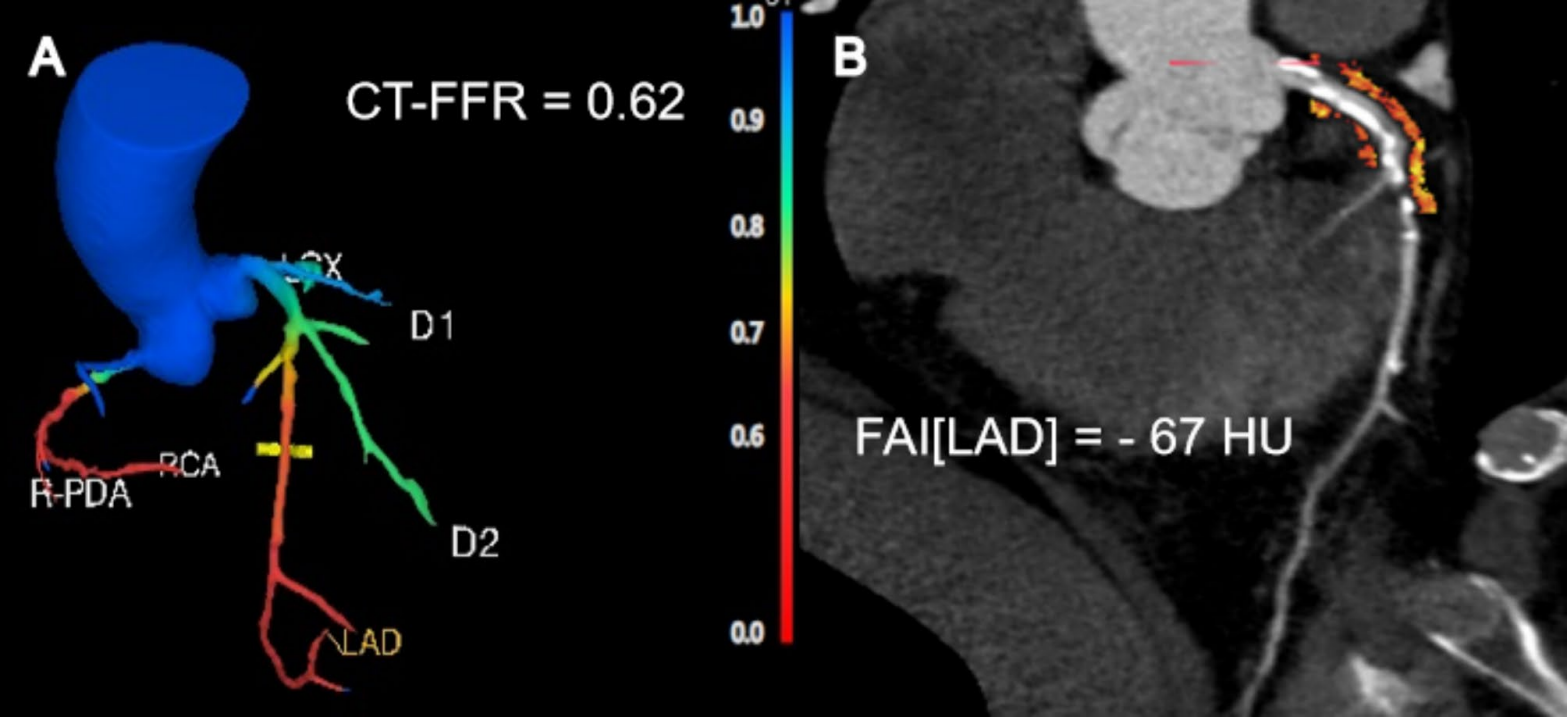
A representative case of CT-FFR and FAI assessment. (A) Three-dimensional color-coded mesh reveals hemodynamically significant lesions in the LAD, RCA, and PDA (CT-FFR = 0.62, CT-FFR ≤ 0.80 is considered positive). (B) Perivascular FAI phenotyping from CCTA in the proximal 0–40 mm of the LAD (FAI[LAD] = − 67 HU, FAI ≥ 70.1 is considered positive). CT-FFR, CT-derived fractional flow reserve; FAI, fat attenuation index; LAD, left anterior descending artery; RCA, right coronary artery; PDA, posterior descending artery; CCTA, coronary computed tomography angiography
